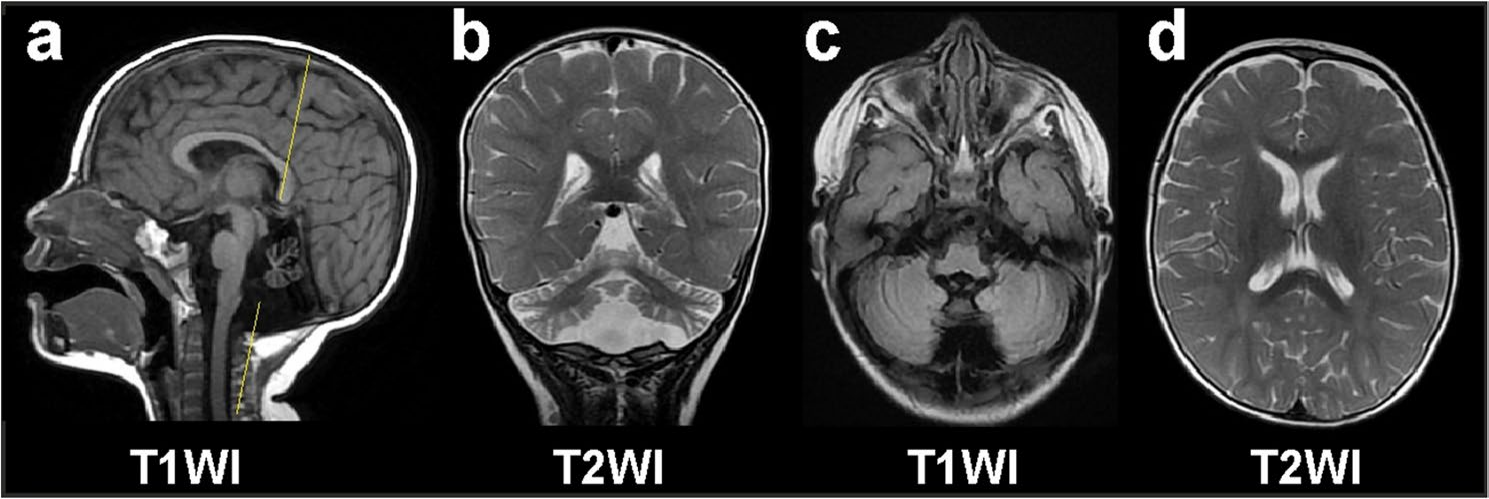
MRI of the head of a 1.8-year-old proband. a hypoplasia of the cerebellar vermis (sagittal plane); b, c: hypoplasia of the cerebellar hemispheres, thinning of the cerebellar cortex; and d: parieto-occipital periventricular areas of decreased myelination
